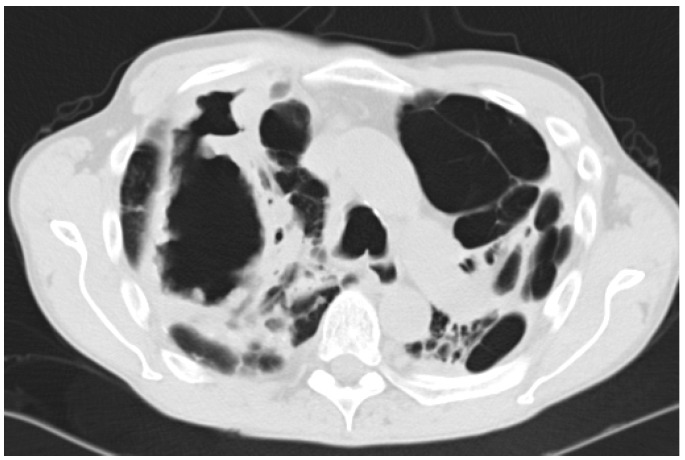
Identification of co-existent pathologies: CT scan from a patient with CCPA, *M. avium intracellulare* and severe bullous emphysema.
